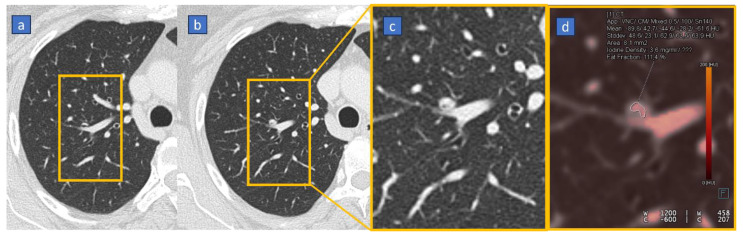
Fifty-one-year-old woman with surgically proven endobronchial tumor. On the baseline high resolution CT scan, (a) there is no evidence of tumor. During the one year follow up study, (b) there is evidence of a tiny endobronchial filling defect. On the corresponding contrast enhanced cropped axial image (corresponding to yellow box), (c) it is not possible to distinguish between a mucoid plug and a solid tumor. On the corresponding contrast enhanced DECT iodine map image, (d) it is possible to demonstrate a subtle iodine uptake, confirmed on the quantitative assessment achieved by placing a free hand ROI on the tumor.
